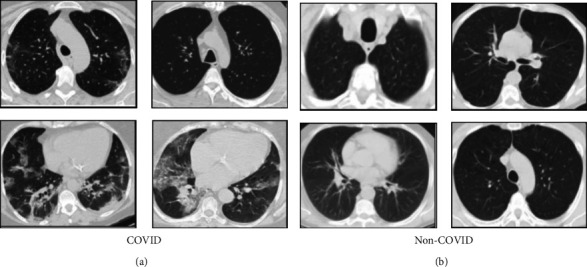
Sample CT slices from the dataset (a) for COVID cases and (b) for non-COVID cases.
